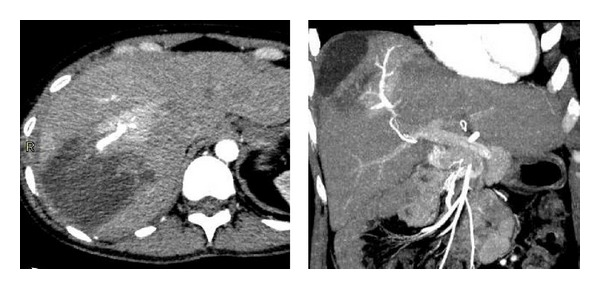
Follow-up CT performed 13 days after the injury. Enhanced CT reveals an arterioportal fistula in segment 8 of the liver with partial enhancement of the liver parenchyma in the early phase.
